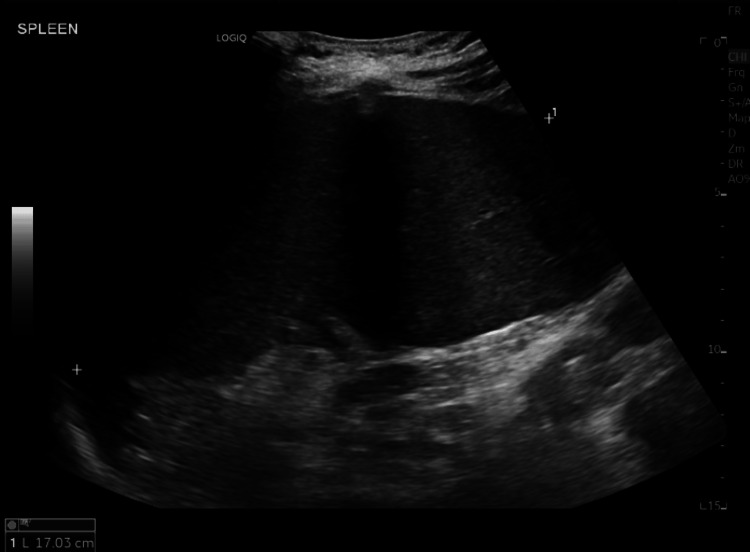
An ultrasound hepatic vein doppler showed splenomegaly of about 17 cm
